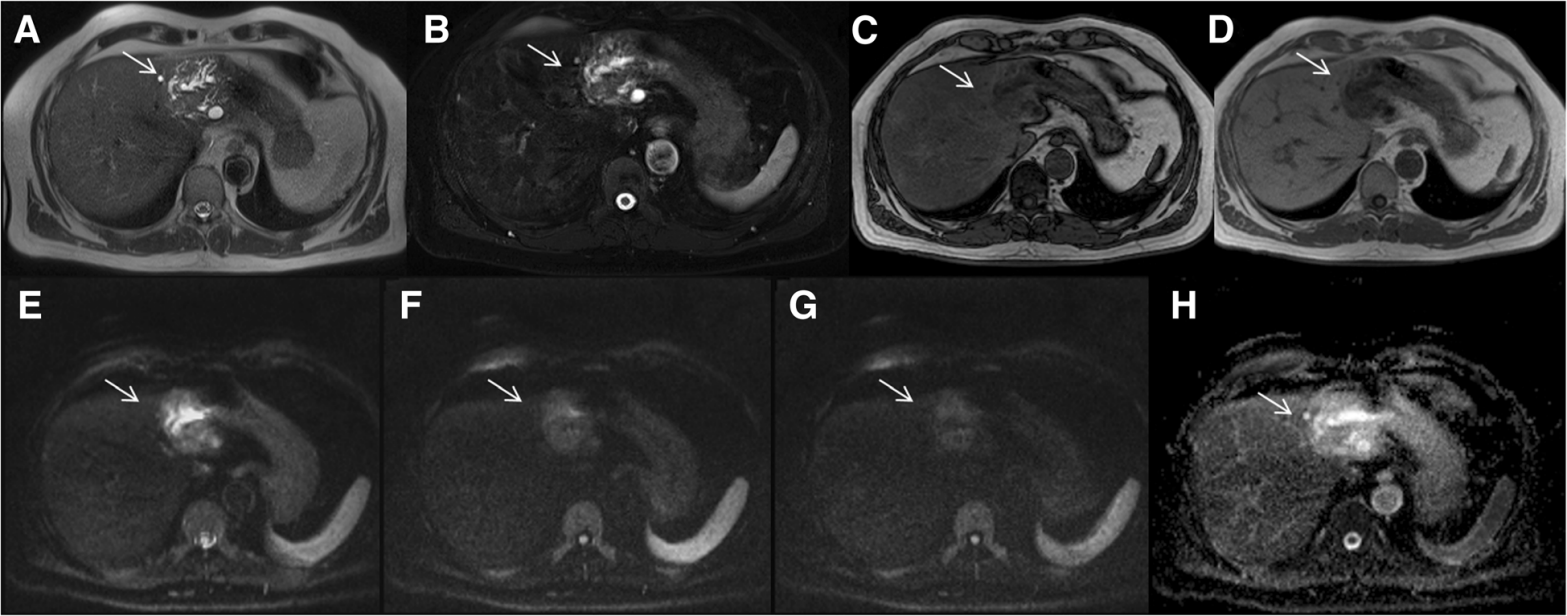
The same patient of Fig. 6. The peribiliary metastasis (arrow) appears hyperintense in T2-W (a and b; see also the biliary tree dilatation due to the lesion), hypointense in T1-W (c and d: T1-W out-in phase sequences) with restricted diffusion for all b values in DWI (e: b50 s/mm^2^; f: b600 s/mm^2^, g: b800 s/mm^2^; h: ADC map). The ADC value is 1.31 × 10–3 mm^2^/s
